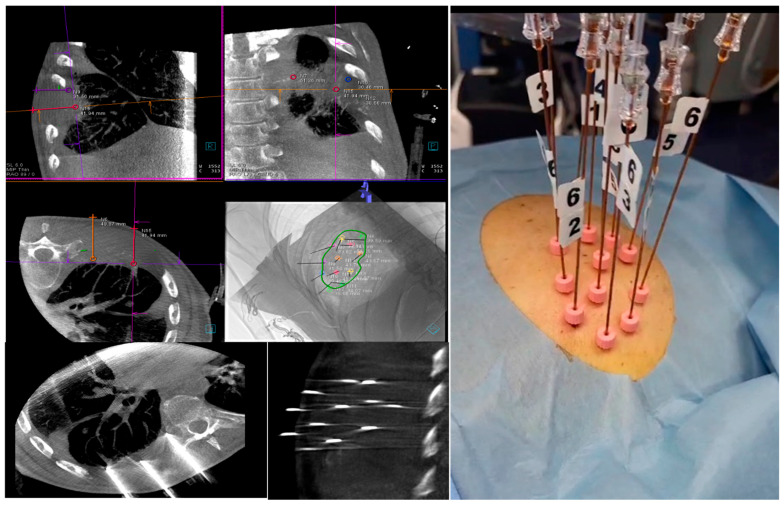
ECT planning and needle positioning under CBCT guidance.
